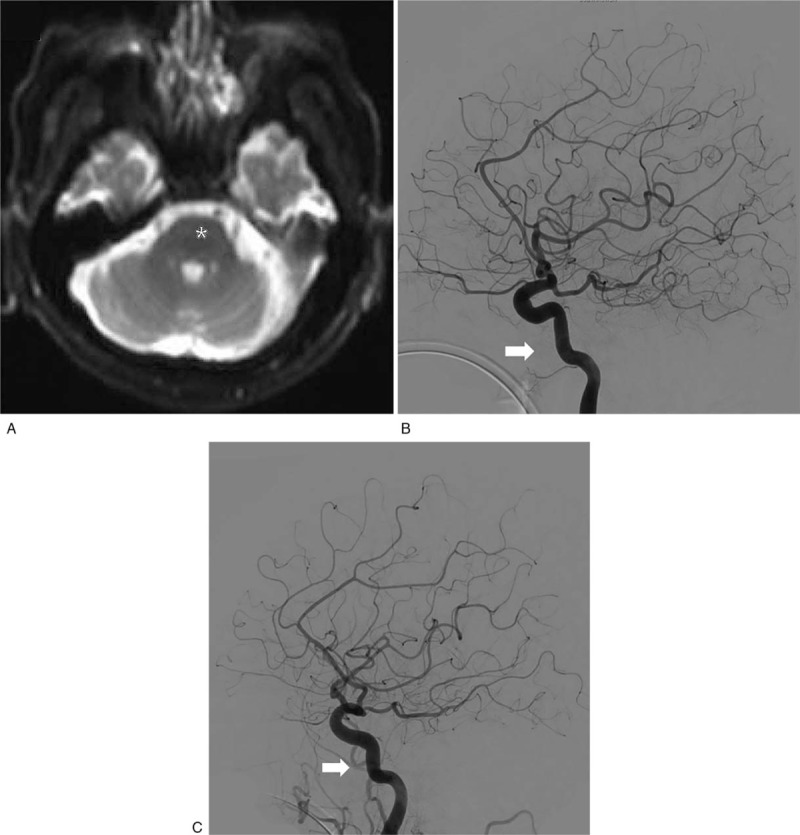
A case of nontraumatic cerebral fat embolism in an old man (case 2) that presented with distal basilar artery infarction. (A) Diffusion-weighted magnetic resonance imaging reveals very subtle hyperintensities in the right ventral pons and right medial longitudinal fasciculus (asterisk). (B) Trans-femoral cerebral angiography reveals short segmental occlusion in the distal basilar artery trunk and V4 segment of the left vertebral artery (white arrow). (C) Post-interventional angiography reveals almost complete recanalization of the occluded areas (white arrow).
